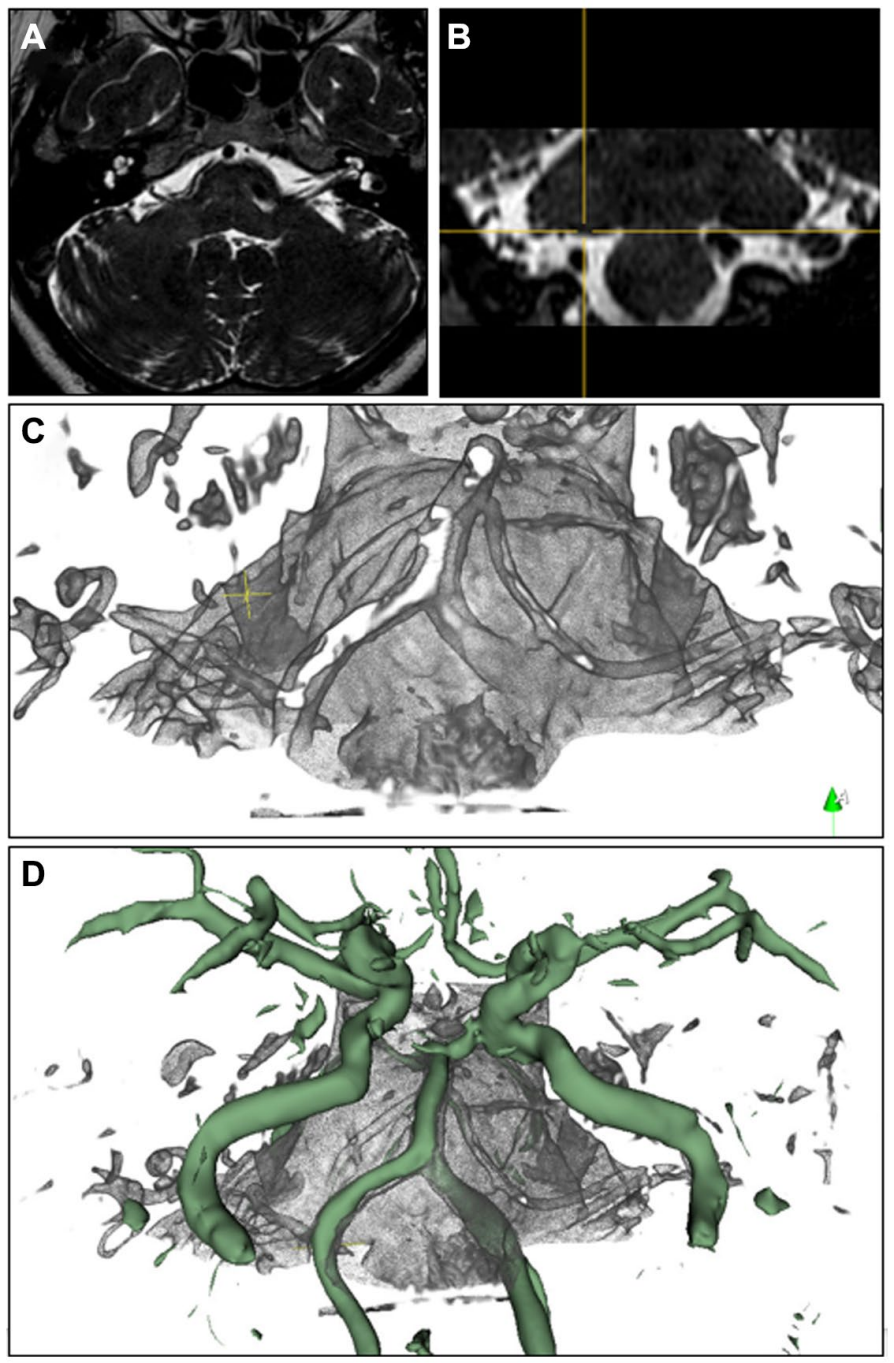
Rendering image of NSIWR. (A,B) MR images, (C) NSIWR in 3d space, (D) NSIWR and fusion with MRA reconstruction. The green vessels are the segmented reconstructions from MRA.
